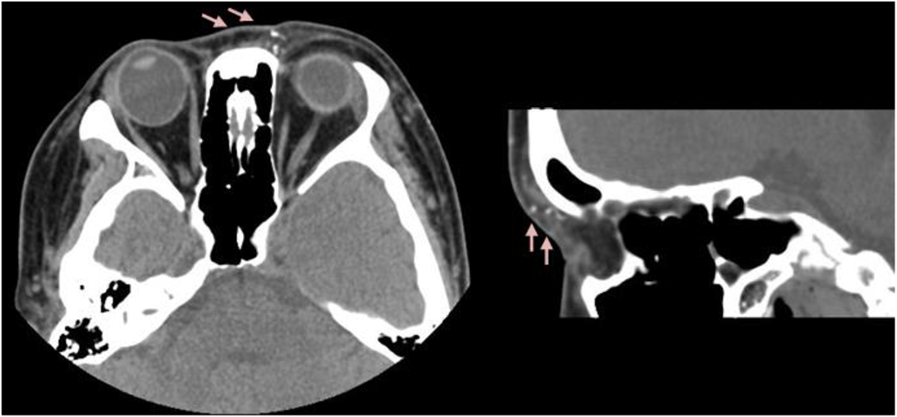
Orbital CT demonstrates multiple radiopaque spots in the subcutaneous layer of medial aspect of left periorbital region, suggesting CaHA deposition.
